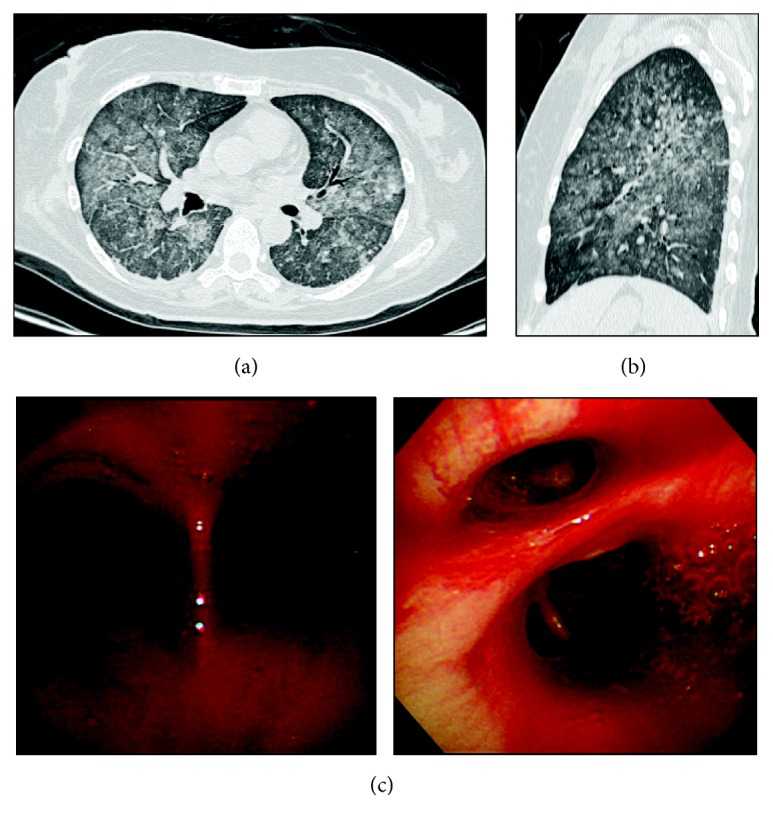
Imaging studies. Axial (a) and sagittal (b) chest computed tomography scans showing diffuse ground-glass opacities bilaterally. Bronchoscopy images (c) showing bloody discharge around the bifurcation of the trachea and the right intermediate and inferior bronchi.
